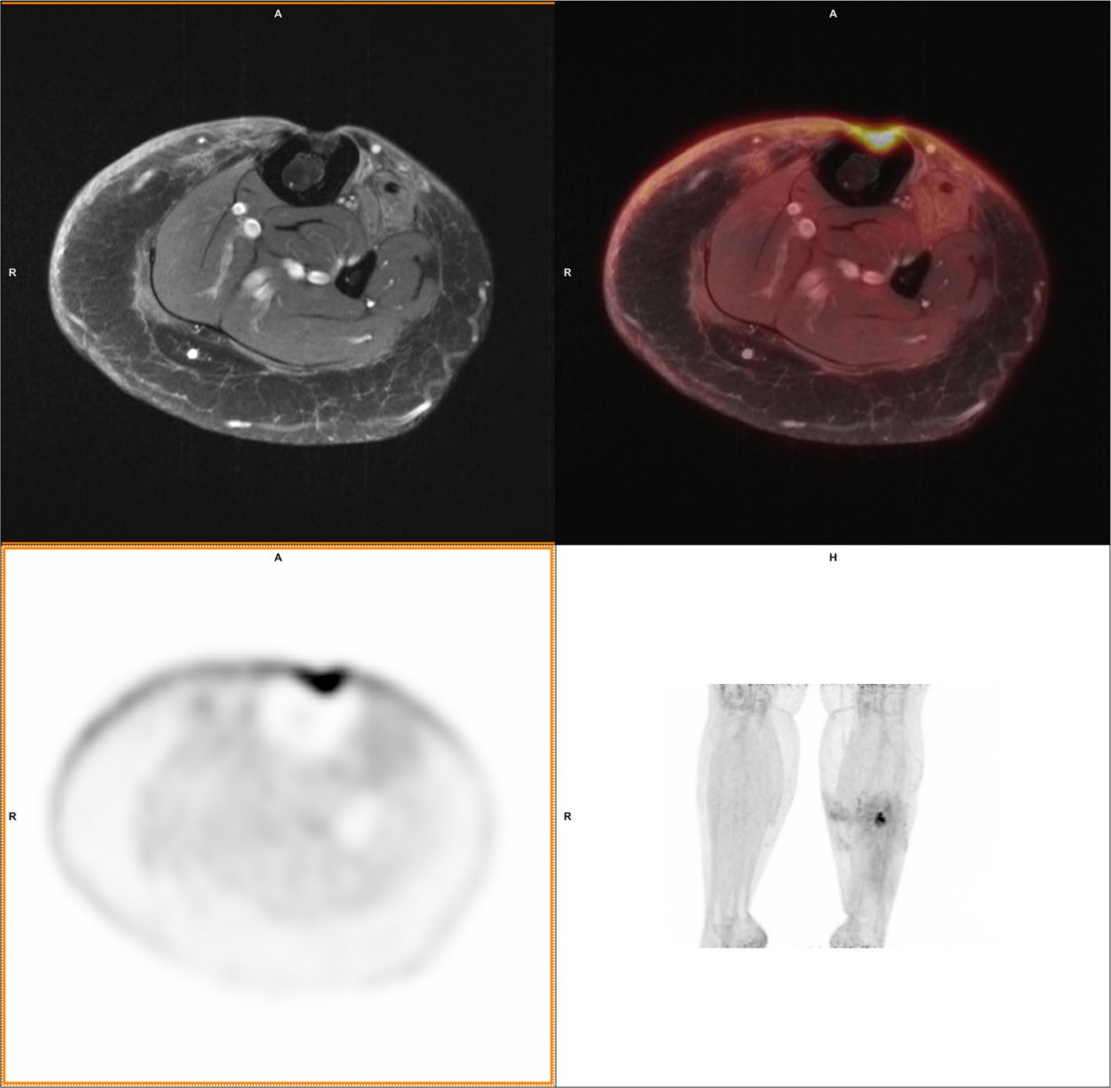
A 33 year old woman with a 2 year old soft tissue trauma as a suspected contiguous source of osteomyelitis in the tibia. FDG accumulation was measured in the defect, and the cortex was assessed to be involved based on the PET scan. A lack of signs for oedema and (aside from the pre-existing defect) a seemingly intact cortex on MRI resulted in a consensus PET/MRI diagnosis of soft tissue infection. This was contradicted by the final clinical diagnosis (osteomyelitis) based on microbiology and clinical follow-up
